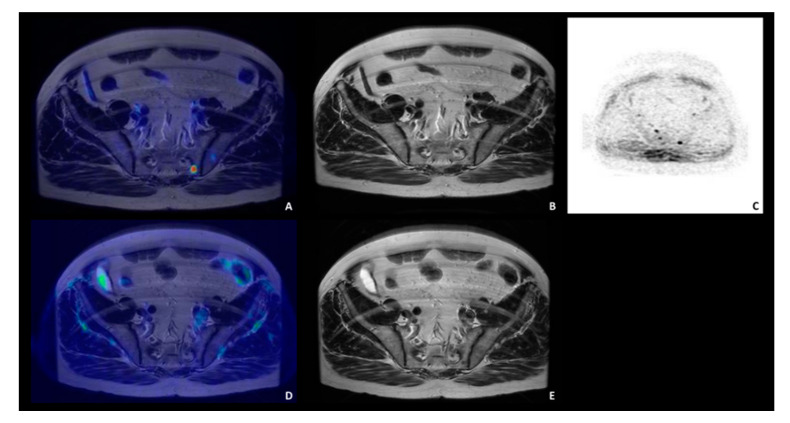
A 59 years-old patient with biopsy-proven PCa (patient n. 6), Gleason score 9 (4 + 5) with a PSA level at diagnosis of 11.0 ng/mL. ^68^Ga-PSMA PET/MRI (top panel; (A): ^68^Ga-PSMA PET/MRI; (B): axial T2-weighted sequence of the pelvis; (C): axial DWI (b = 1000) displayed with inverted greyscale map) showed increased uptake in correspondence of the left sacral ala, where MRI detected a bone metastasis; ^68^Ga-DOTA-RM2 PET/MRI (bottom panel; (D): ^68^Ga-DOTA-RM2 PET/MRI; (E): axial T2-weighted sequence of the pelvis) did not show any ^68^Ga-DOTA-RM2 in correspondence of the bone metastases.
